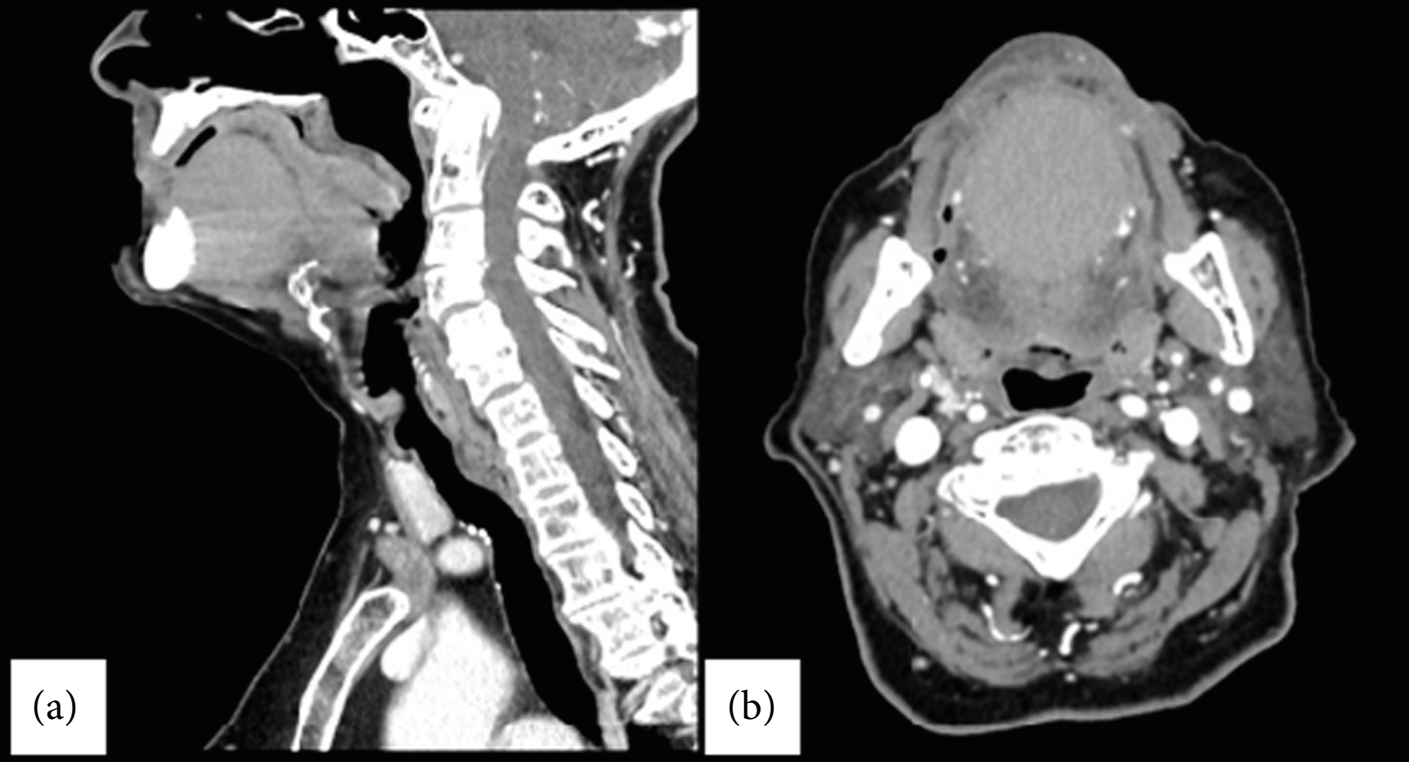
CT scan in (a) sagittal and (b) axial sections that highlight a rounded neoformation located at the level of the oral floor, not absorbing the contrast medium.
